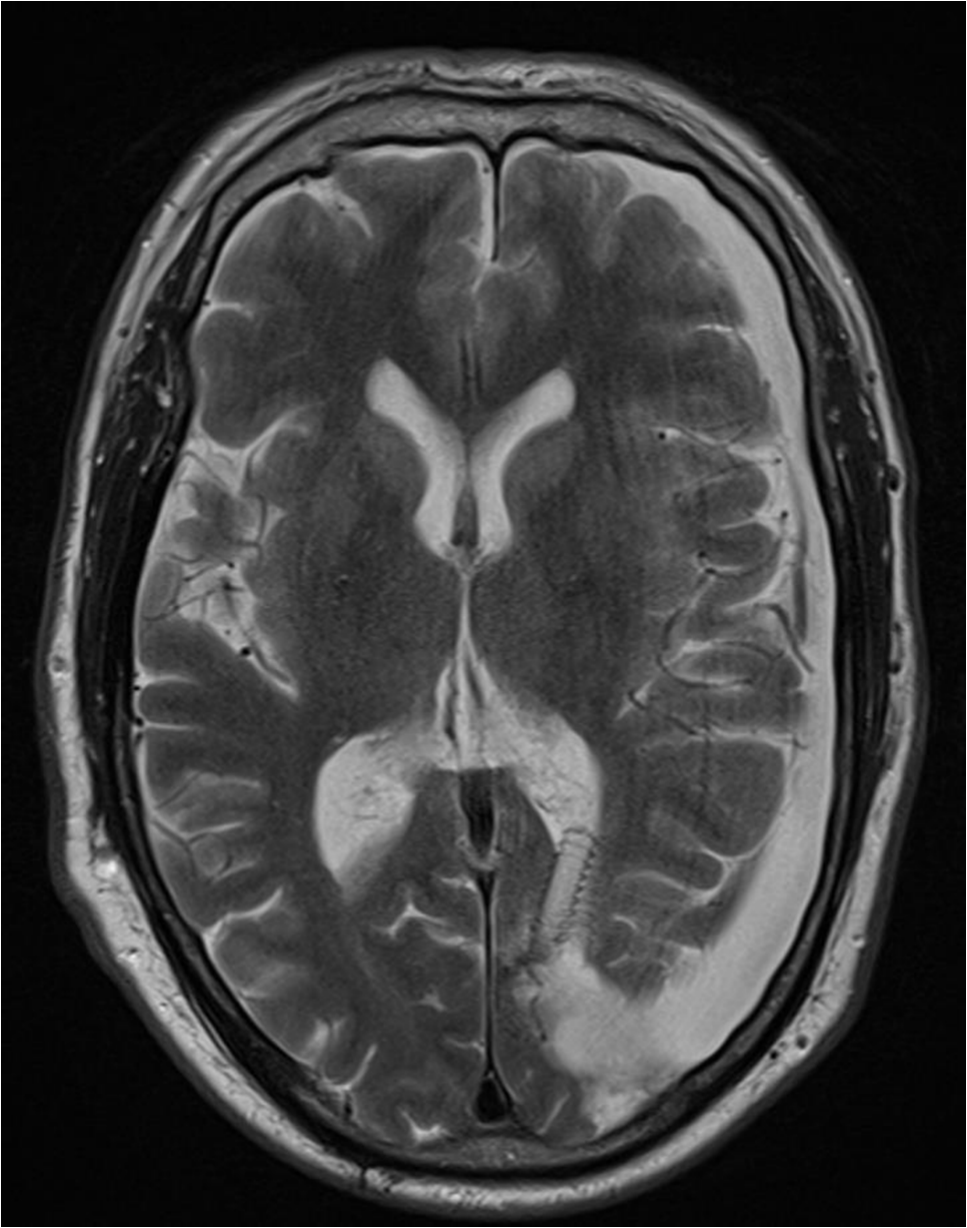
Postoperative SWI-weighted axial MRI showing the ringed wall structure of the vascular graft within the slit valve
